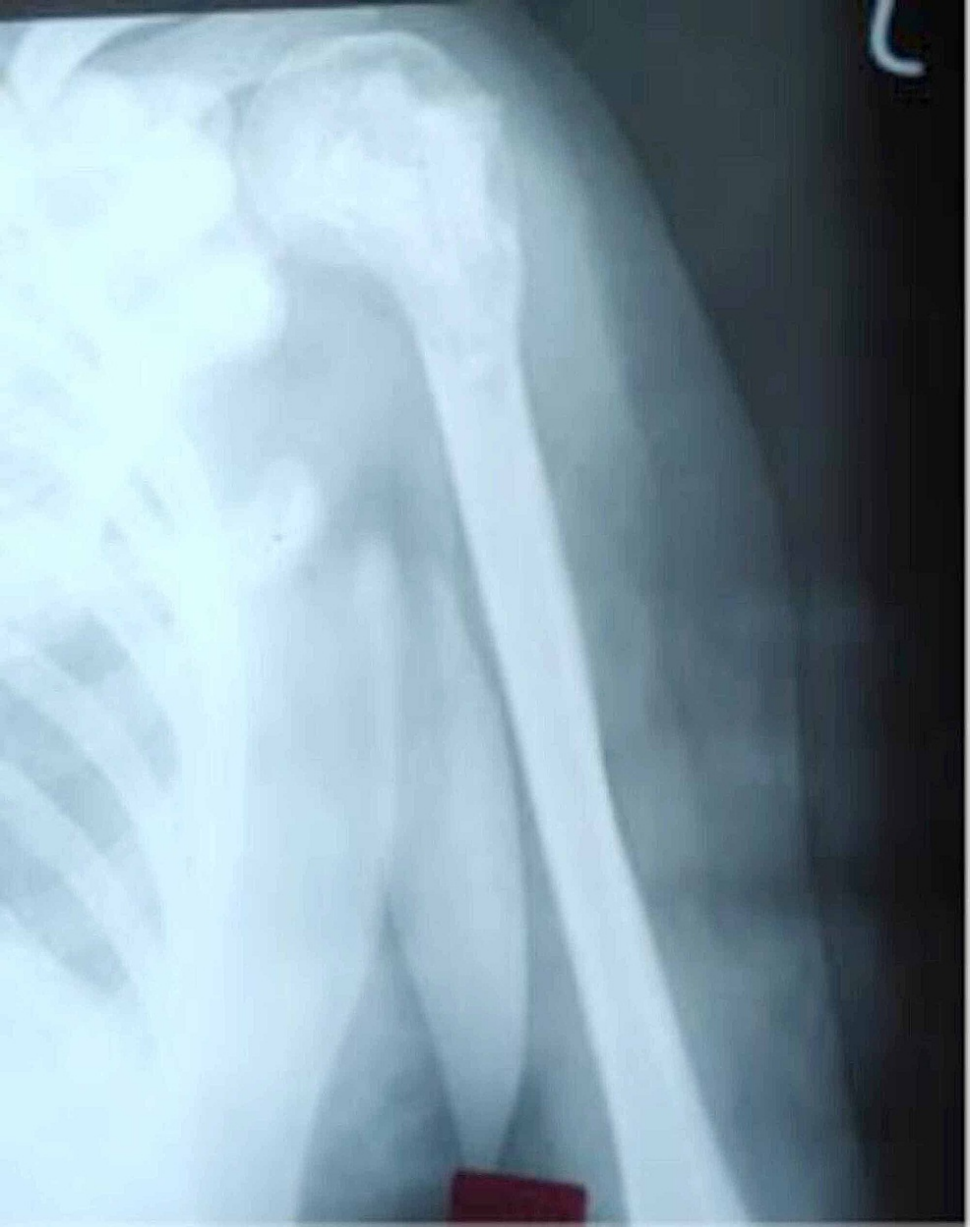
Postoperative X-ray following curettage and bone grafting (2001)
